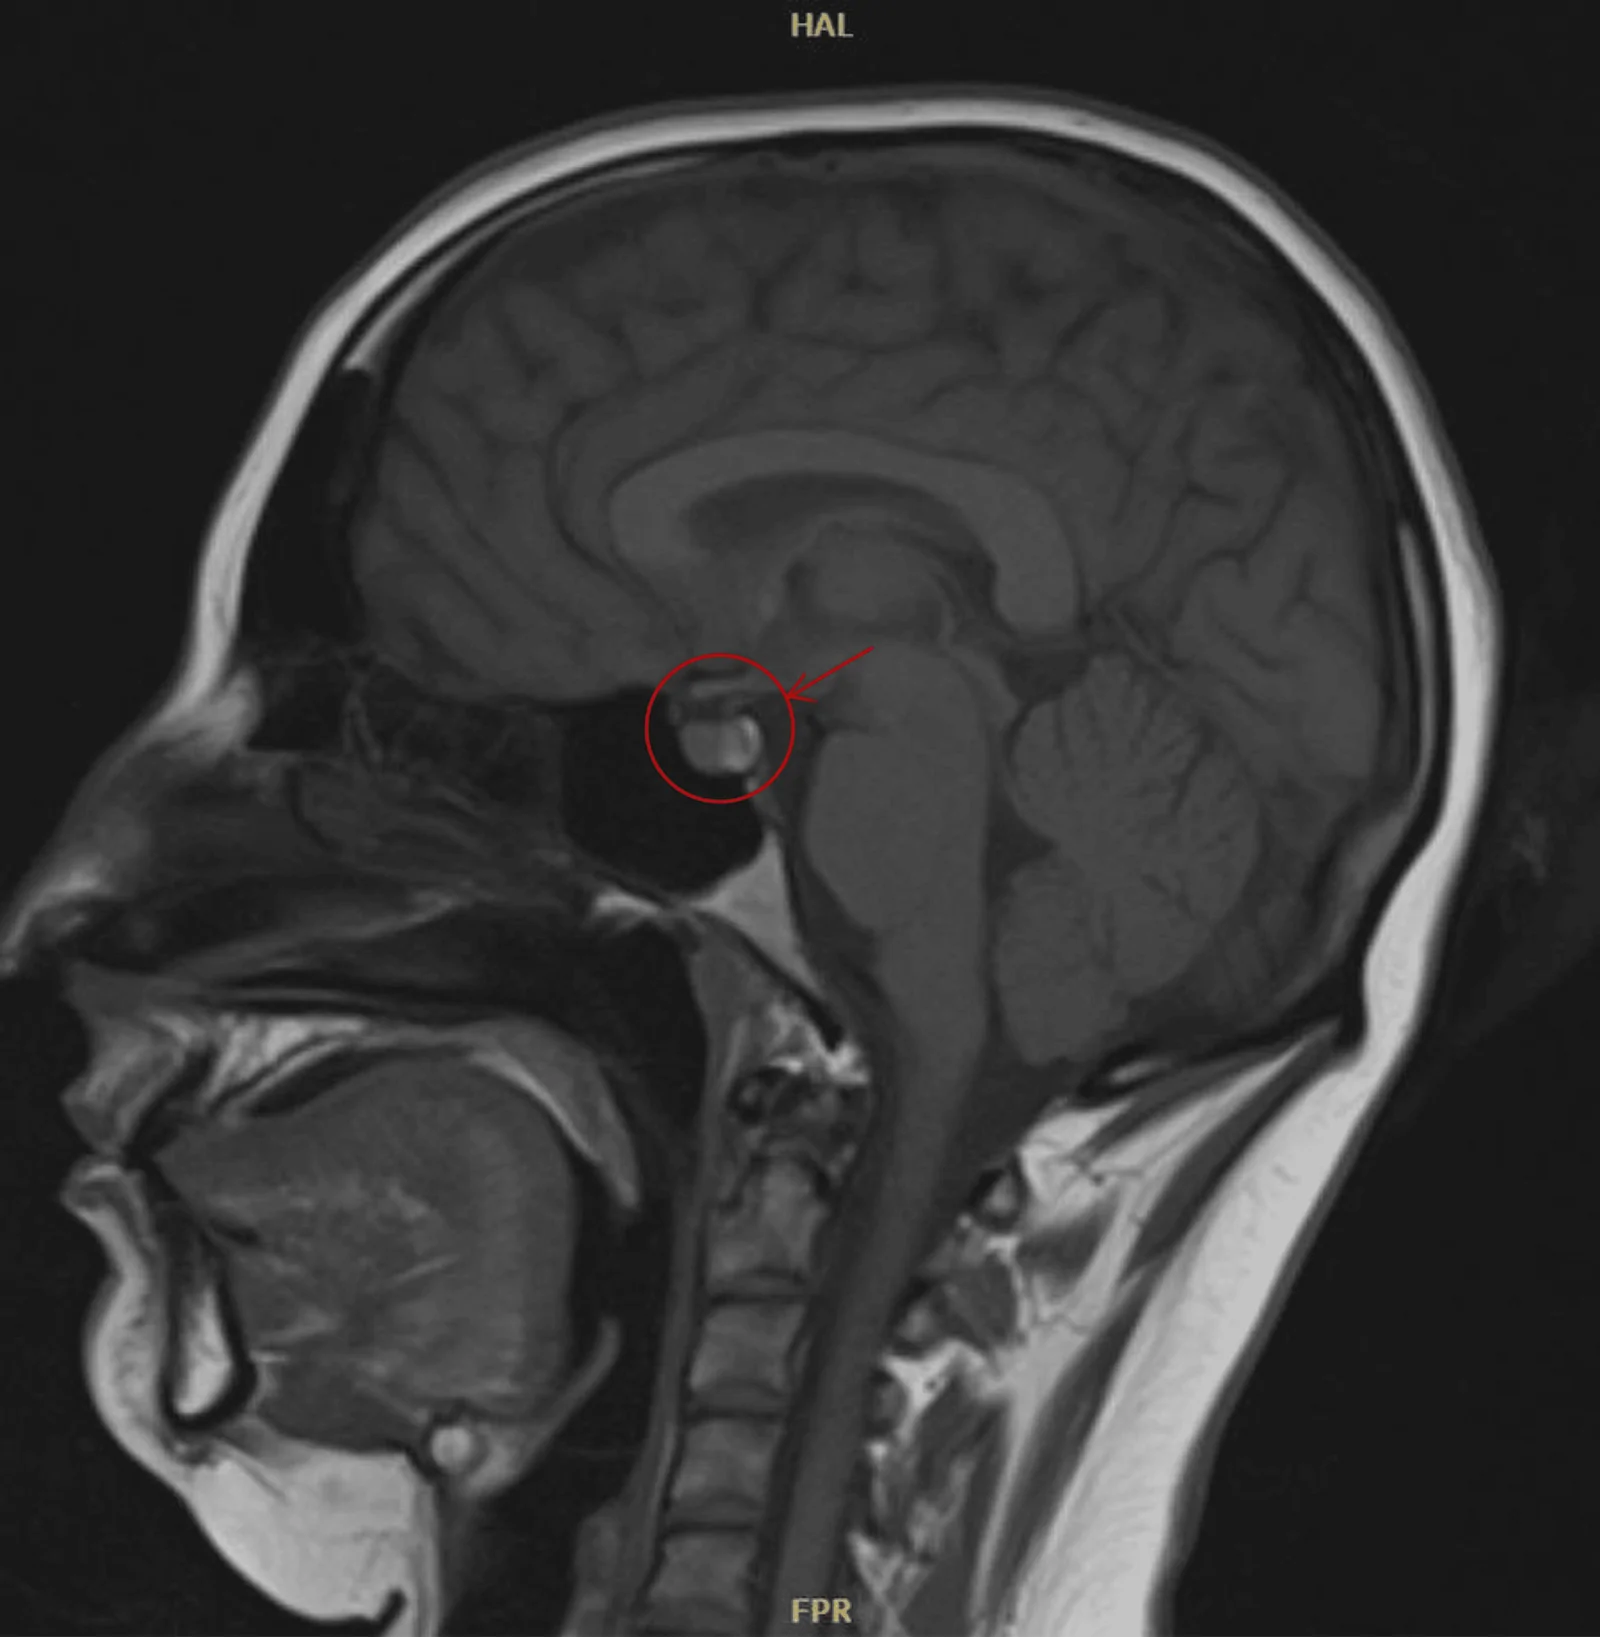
Magnetic resonance imaging findings Magnetic resonance imaging of the head demonstrates a lesion with high signal intensity on T1-weighted imaging and low signal intensity on T2-weighted and fluid-attenuated inversion recovery imaging, without restricted diffusion. The imaging differential diagnoses include calcification and chronic haemorrhage.
